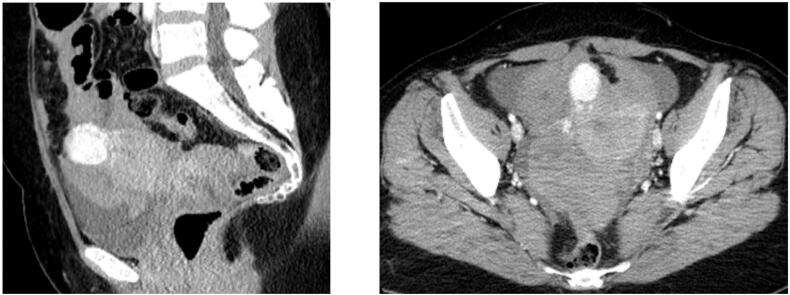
CT imaging showing the uterine tumor with an active bleeding area.
